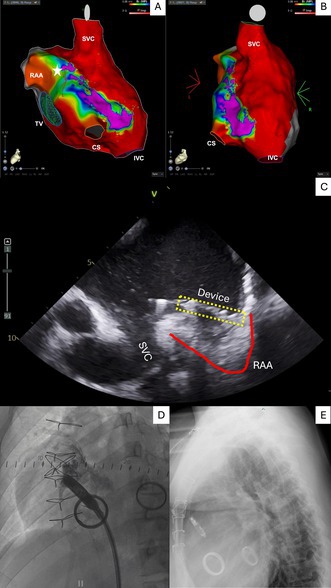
A 60‐year‐old woman with a history of rheumatic heart disease, mechanical mitral and tricuspid valve replacement, multiple catheter ablation procedures for atrial tachyarrhythmias, and prior bi‐atrial Maze surgery presented with recurrent presyncope and near‐syncope. Ambulatory monitoring demonstrated paroxysmal atrial fibrillation with conversion pauses of up to 6 s, consistent with symptomatic sinus node dysfunction. Baseline junctional bradycardia further limited the use of additional rate‐controlling or antiarrhythmic therapy. The patient was maintained on chronic warfarin therapy. Transthoracic echocardiography demonstrated preserved left ventricular systolic function and normally functioning prosthetic mitral and tricuspid valves, without evidence of structural abnormalities that would otherwise explain her symptoms. Given the recurrent symptomatic pauses and inability to optimize medical therapy, permanent pacing was indicated.
